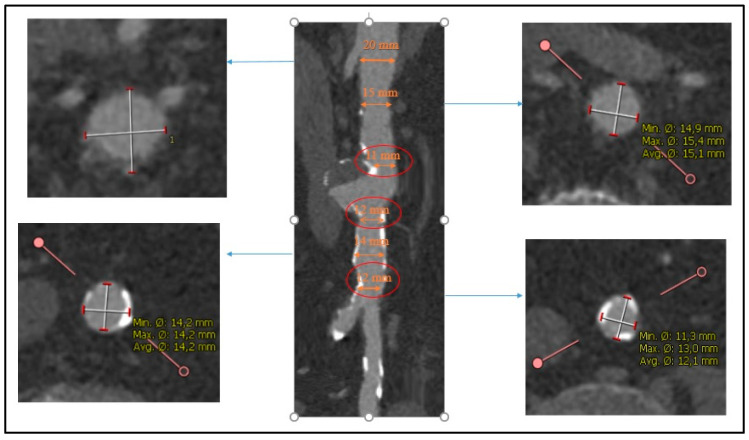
Preoperative computed tomography angiography (CTA) showing saccular aneurysm of the abdominal infrarenal aorta with very narrow and highly calcified aortic bifurcation.
